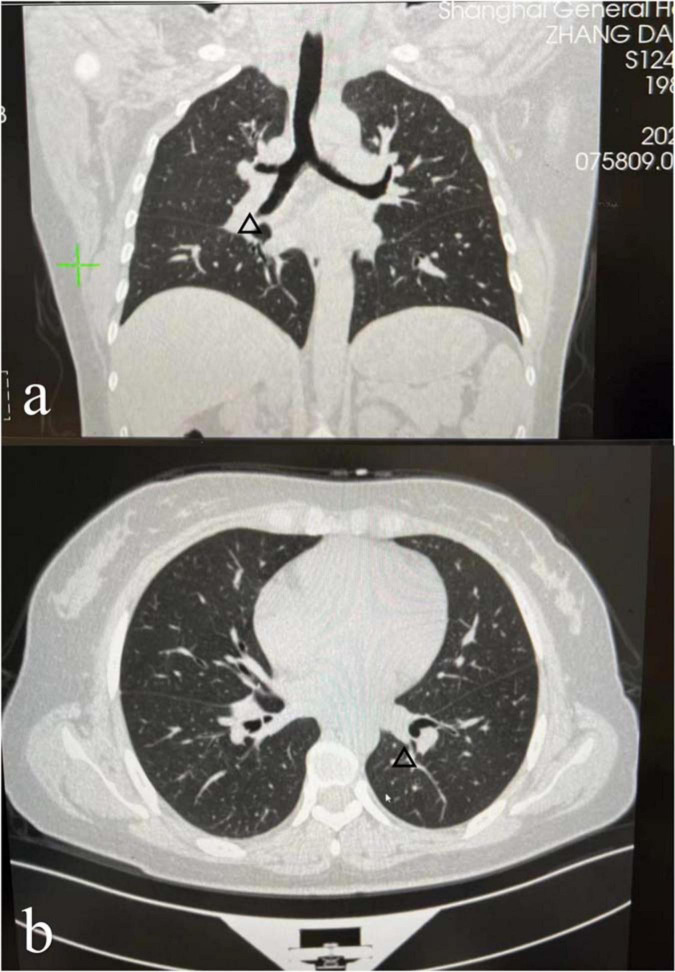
Enhanced computed tomography (CT) of the chest with contrast demonstrated a few diffuse reticulonodular opacities in the bilateral lower lobe, bilateral hilar lymph nodes enlargement [the arrows in panels (a,b)], and in the posterior segment of the right upper lobe are some small ground glass nodules.
